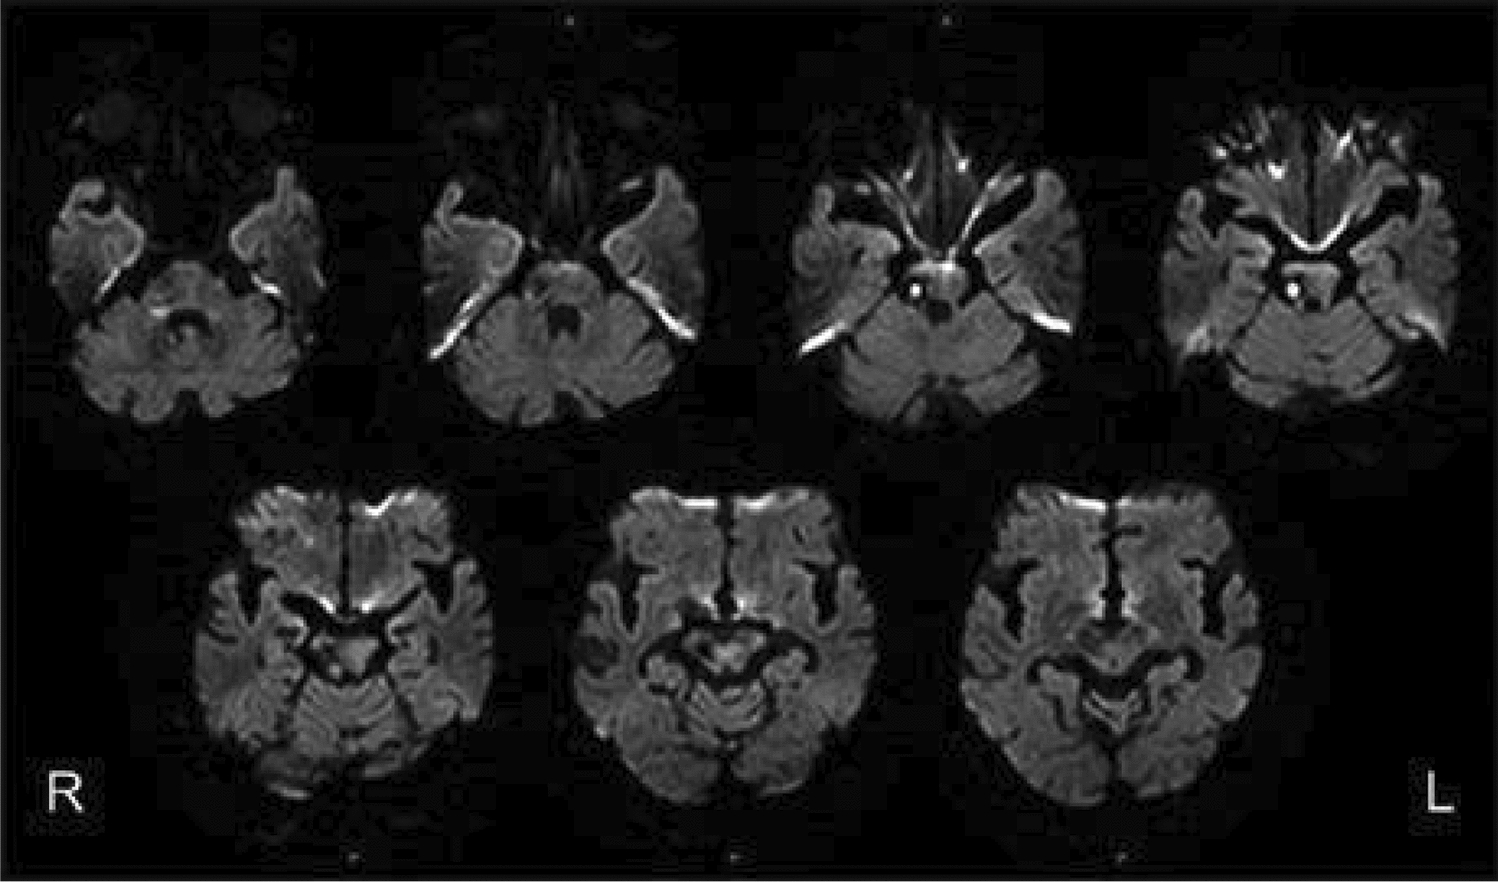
Diffusion-weighted magnetic resonance image showing hemorrhagic foci in the right dorsolateral brain, from the pontine tegmentum to the lower midbrain tegmentum [61]
